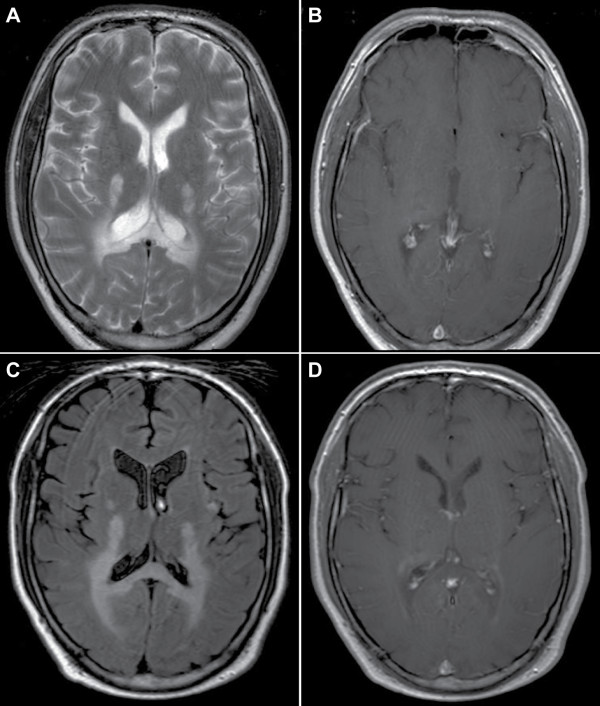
MRI of the brain (T2 (A) and FLAIR (C) images; T1 with gadolinium (B, D)) of a patient with AMN who rapidly deteriorated clinically with new symptoms of cognitive decline. On MRI extensive white matter changes were seen in the parieto-occipital white matter and corpus callosum (A), but no enhancement of the lesion after administration of gadolinium (B). A follow-up MRI about 3 months later shows progression of the white matter lesion (C) and there is now faint enhancement of the rim of the lesion after gadolinium administration (D).
